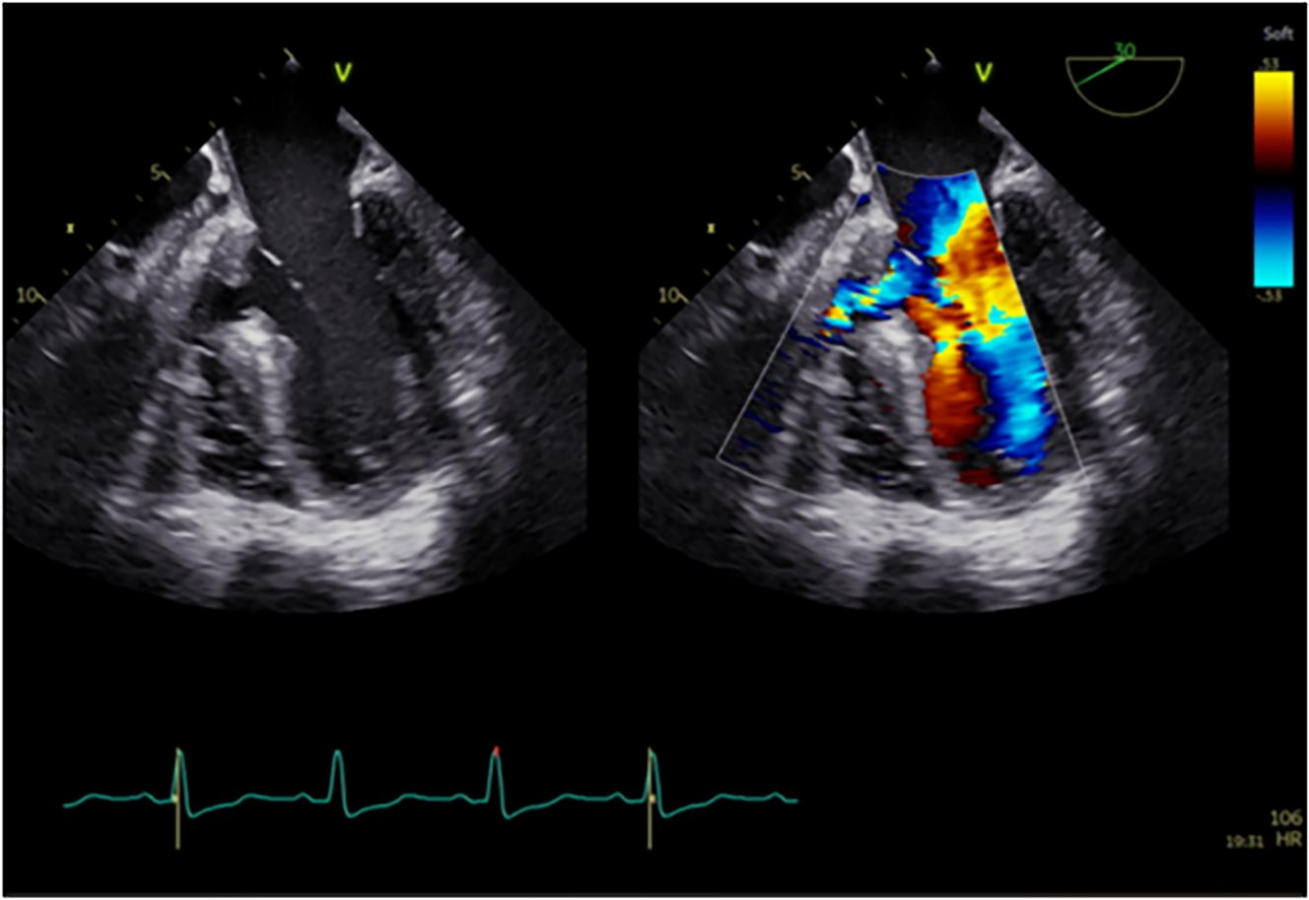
Transoesophageal echocardiography (TEE) revealed a ventricular septal rupture (VSR), measuring approximately 11 mm in diameter, with left-to-right shunting on colour Doppler.
